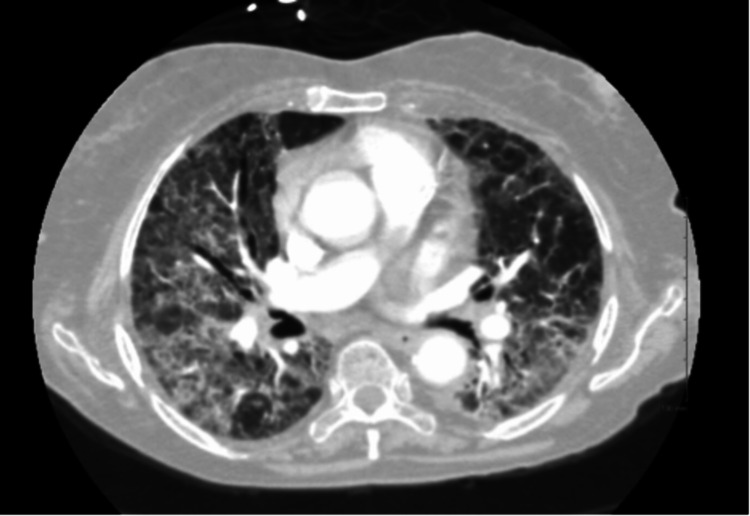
Computed tomography (CT) chest with contrast from initial admission
